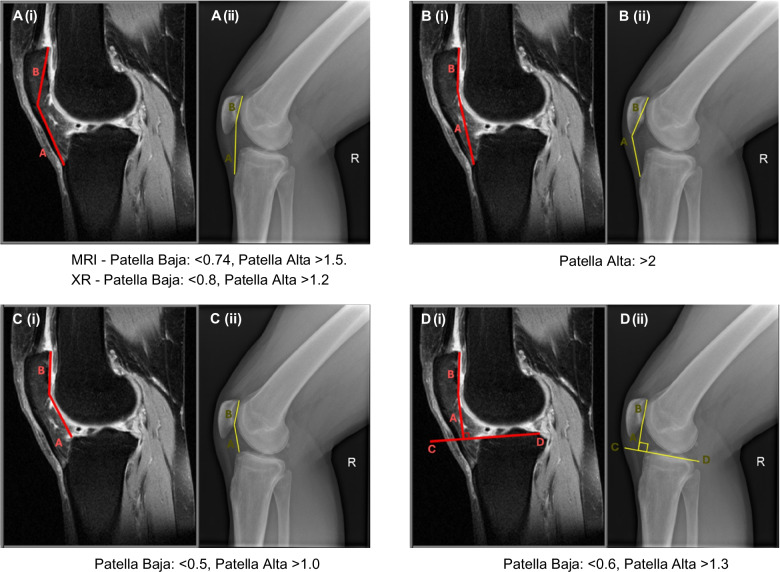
Radiological measurement methodology for patellar height measurements using sagittal MRI and lateral X-ray images. A Insall–Salvati ratio (IS): (i) MRI and (ii) X-ray: IS ratio is patellar tendon length (Line A, from the inferior pole of the patella to the tibial tuberosity) divided by patellar height (Line B, from the inferior to the superior pole of the patella) (Insall and Salvati [22]). B Modified Insall–Salvati ratio (MIS): (i) MRI and (ii) X-ray: MIS ratio is length from the inferior patellar surface to the tibial tuberosity A divided by patellar articular surface height B (Grelsamer and Meadows [23]). C Caton–Deschamps ratio (CD): (i) MRI and (ii) X-ray: CD ratio is distance from the inferior patellar articular surface to the tibial plateau A divided by patellar articular surface height B (Caton and Deschamps [25]). D Blackburne–Peel index (BP): (i) MRI and (ii) X-ray: BP index is distance from the tibial plateau to the inferior patellar articular surface A divided by patellar articular surface height B (Blackburne and Peel [24])
